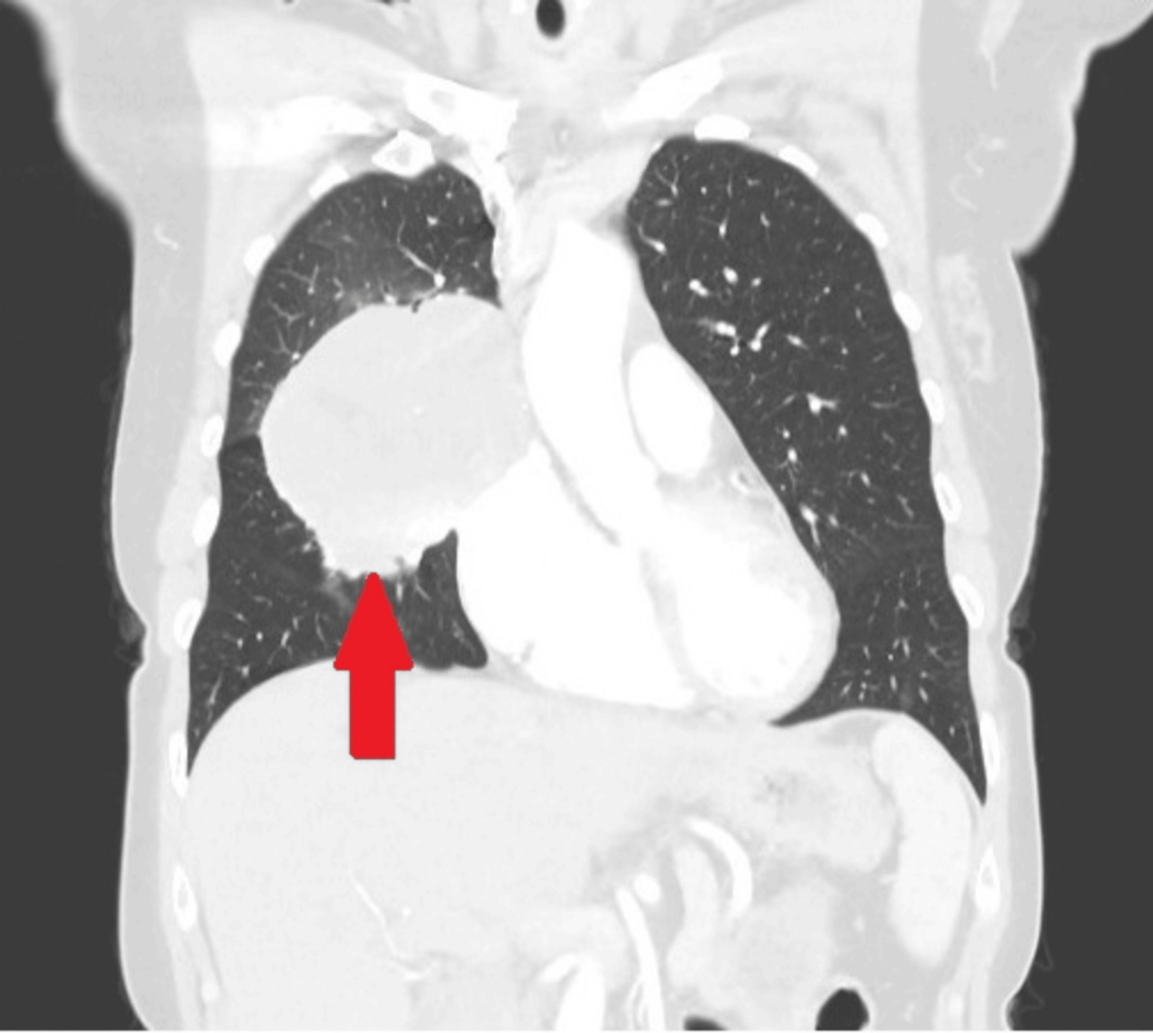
Preoperative CT scan (coronal section) The coronal sections of the CT scan show a large mass in the right upper and middle lobes and its attachment with the mediastinum.
